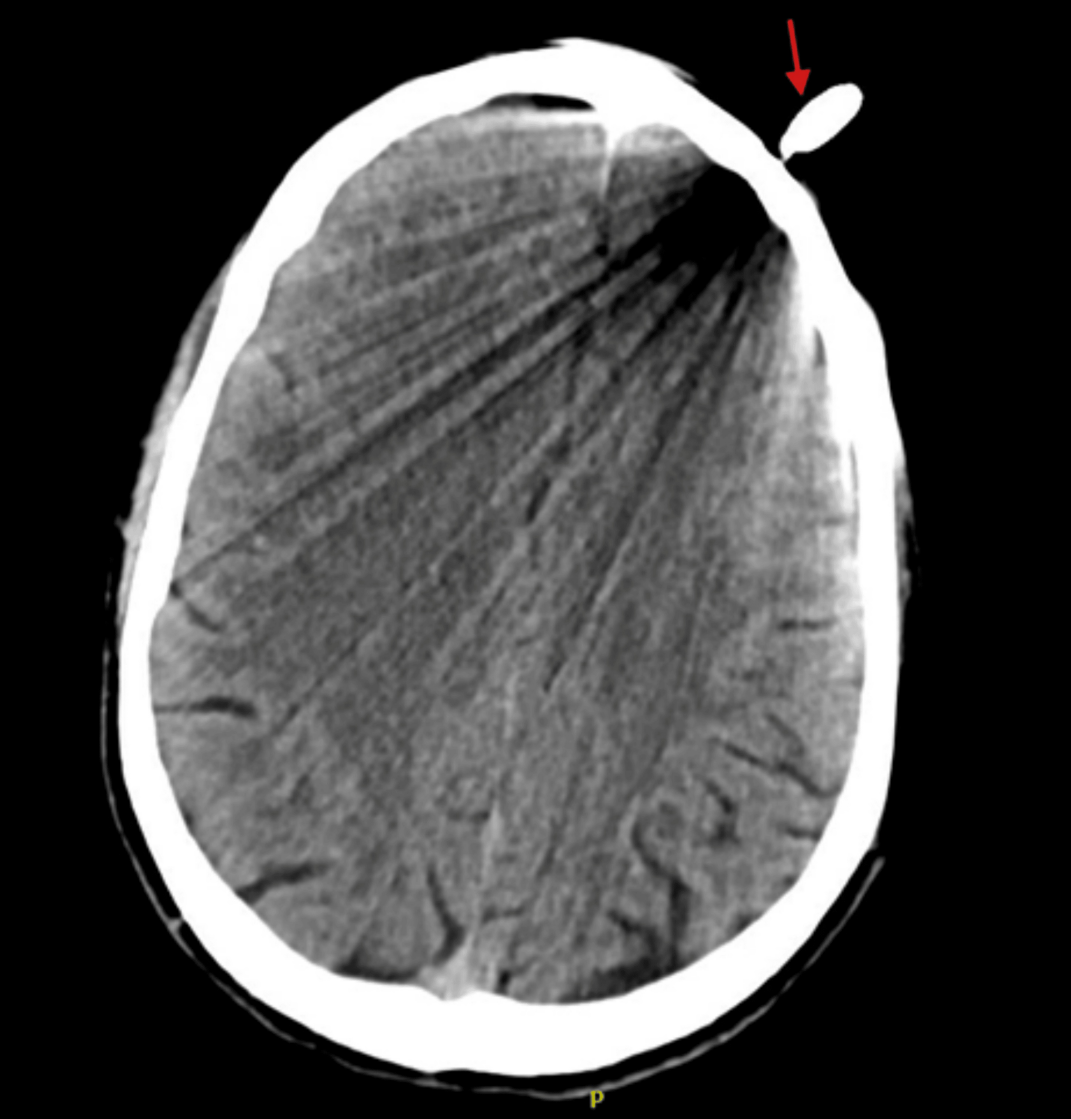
Computed tomography angiogram of the head with and without contrast with brain displaying metallic foreign body embedded within the left frontal cranium (red arrow) with significant beam artifacts
